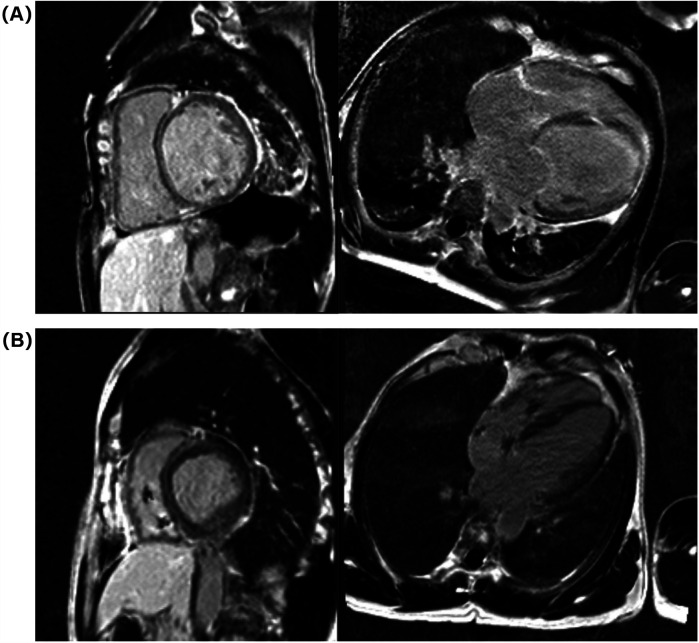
Cardiac MRI images at admission (A) and 1-year follow-up (B).
